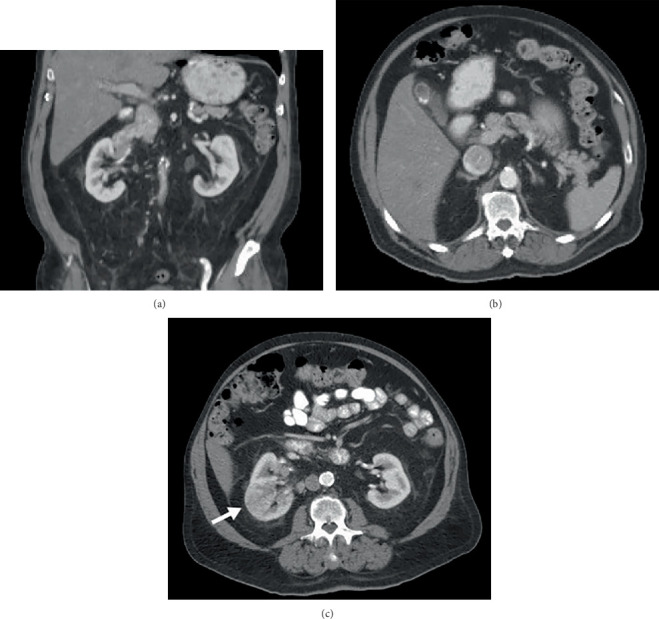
Coronal contrast-enhanced CT (CECT), performed before PSMA PET/CT, in which a right renal mass appears to extend to the right renal vein and IVC (a). The filling defect within the suprarenal IVC (b) and the right renal mass (c, *white arrow*) are also appreciated on axial images.
